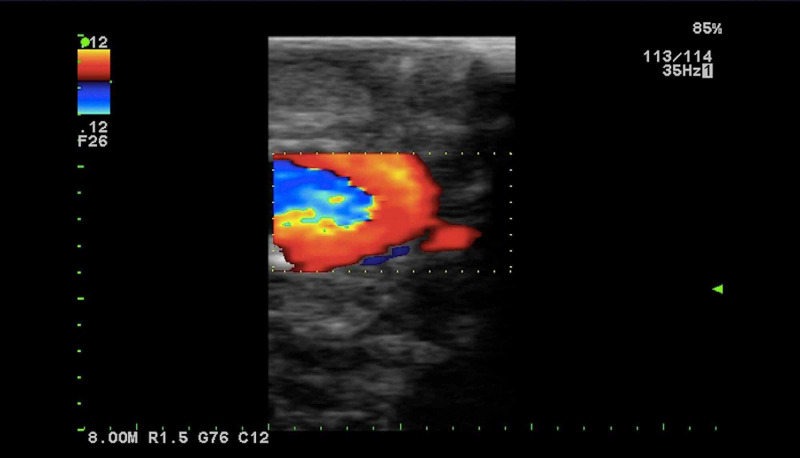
Doppler ultrasonography of the cavernous internal carotid artery
